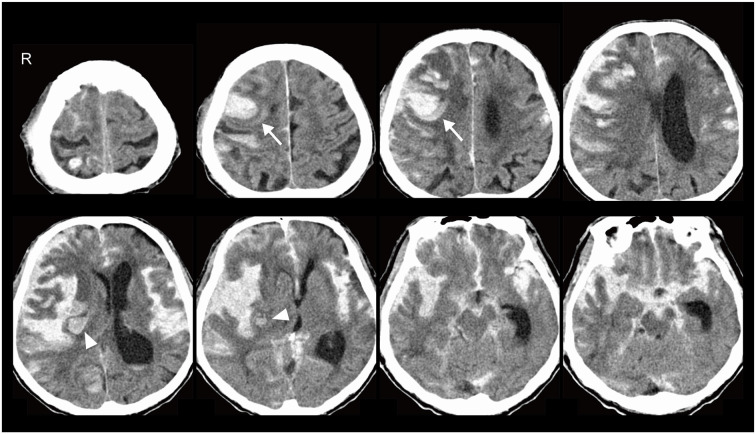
Head CT showed right frontal lobe (arrows) and insula (arrowheads) intracerebral haemorrhage (ICH) adjacent to the brain surface. Blood was also noted in the subarachnoid space, spreading from the basal cistern to the bilateral Sylvian fissure with hydrocephalus and brain herniation.
